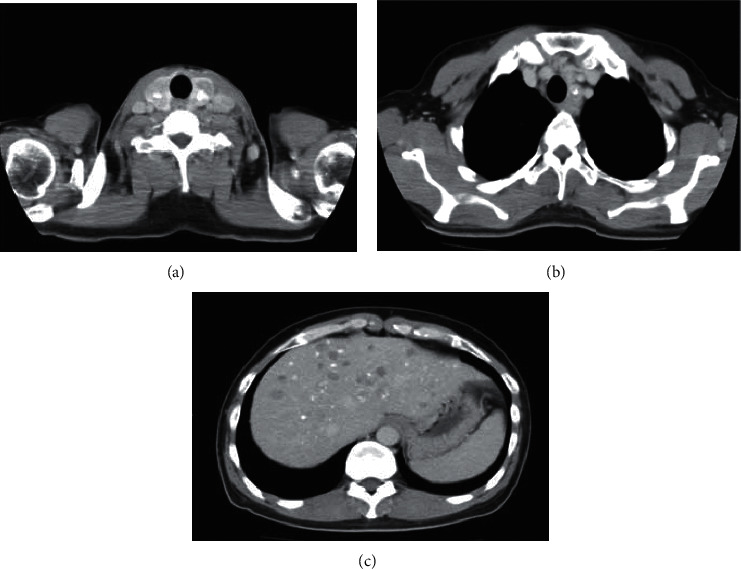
At the first visit to our hospital, computed tomography revealed a thyroid tumor (a), lymph node metastasis (b), and multiple cysts in the liver (c). The tumor was accompanied by calcification.
